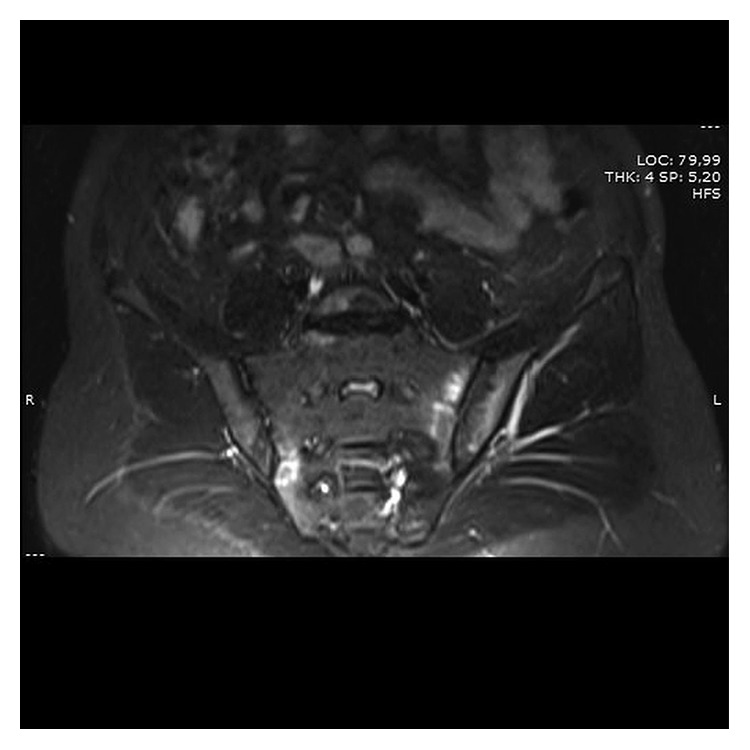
Coronal gadolinium-enhanced fat-suppressed T1-weighted MRI scan of the sacroiliac joints showing chronic degeneration and acute sacroiliitis.
